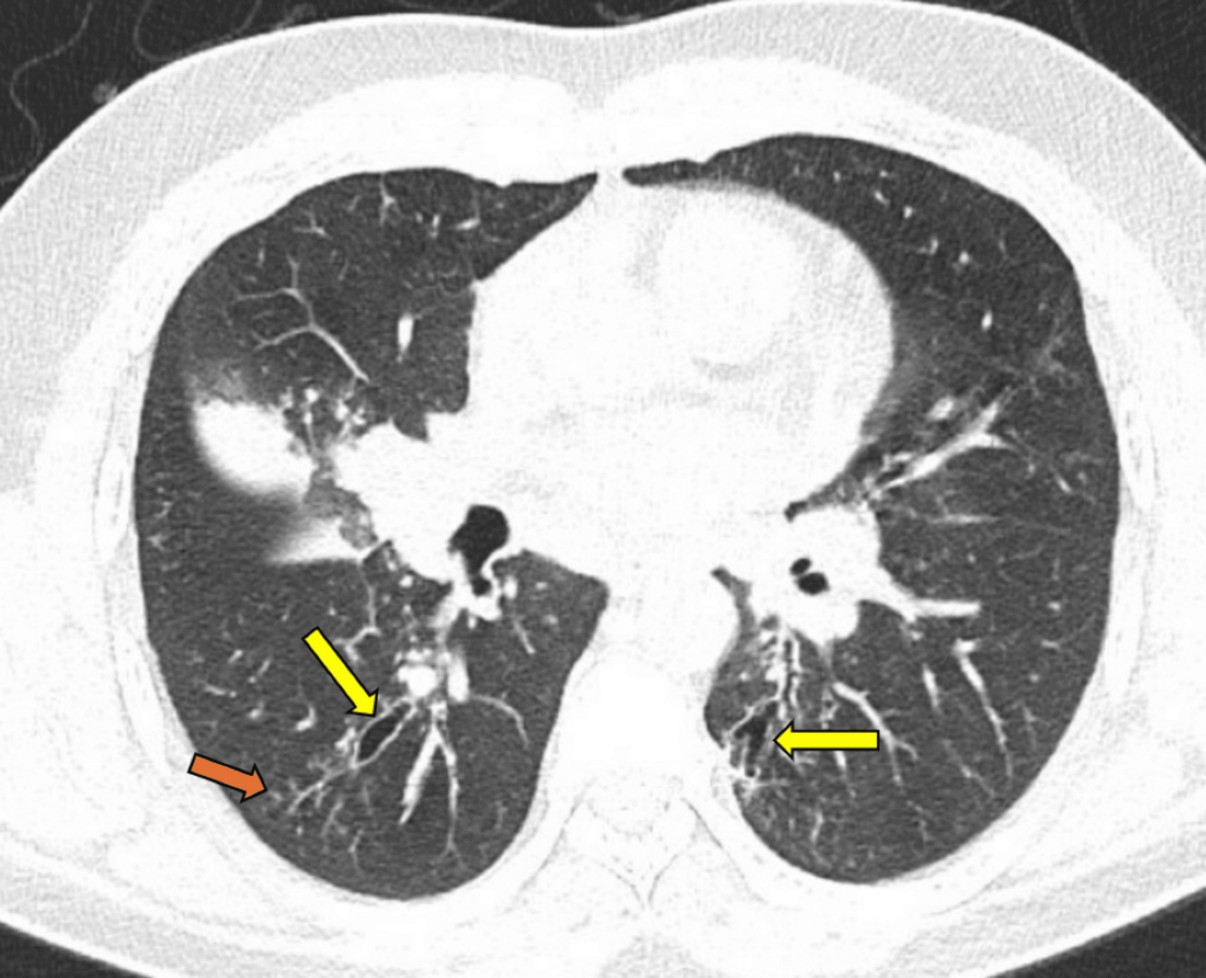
The axial image of the chest CT in the lung window demonstrates bilateral bronchiectasis (yellow arrows), along with small nodular opacities and tree-in-bud changes (orange arrow).
